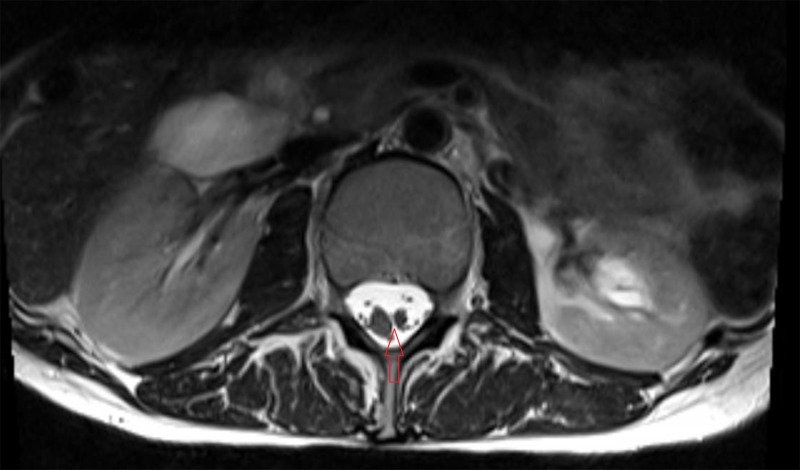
Axial T2 magnetic resonance imaging scan showing a split cord malformation (red arrow)
